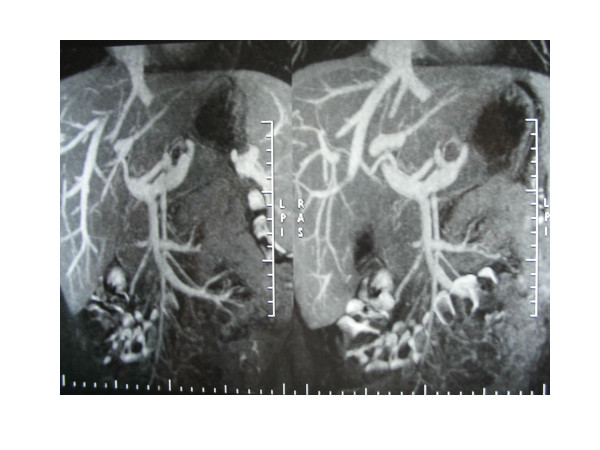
Magnetic resonance imaging. Decrease in the size and number of liver abscesses and no signs of thrombosis.
